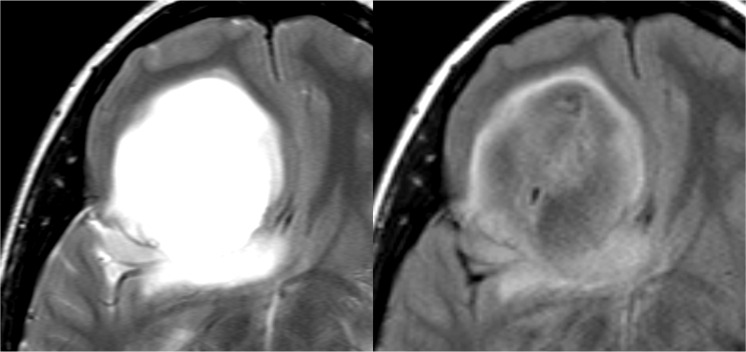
An example of T2-FLAIR mismatch sign. A patient with IDH-mutant astrocytoma in the right frontal lobe. Left shows T2-weighted image and right shows FLAIR image.
